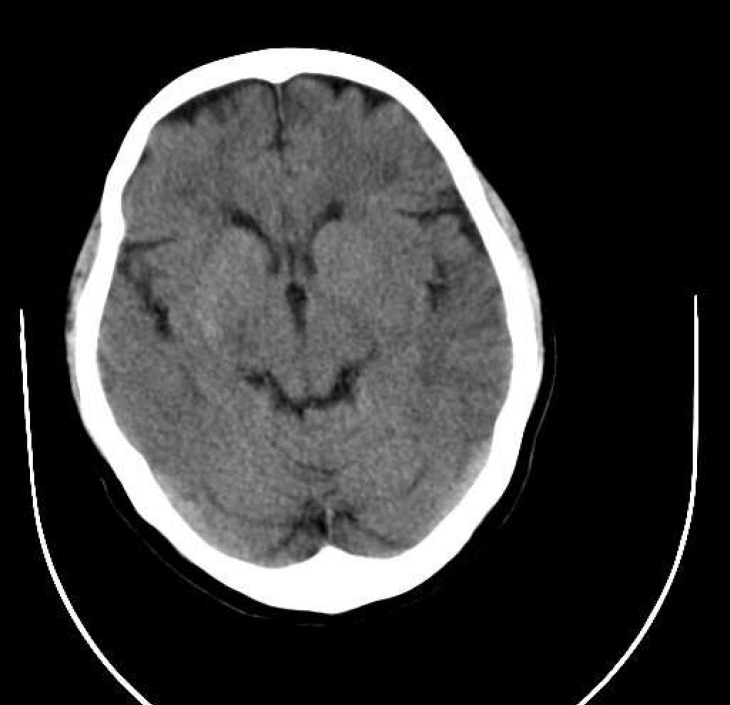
CT scan prior to tissue plasminogen activator administration
